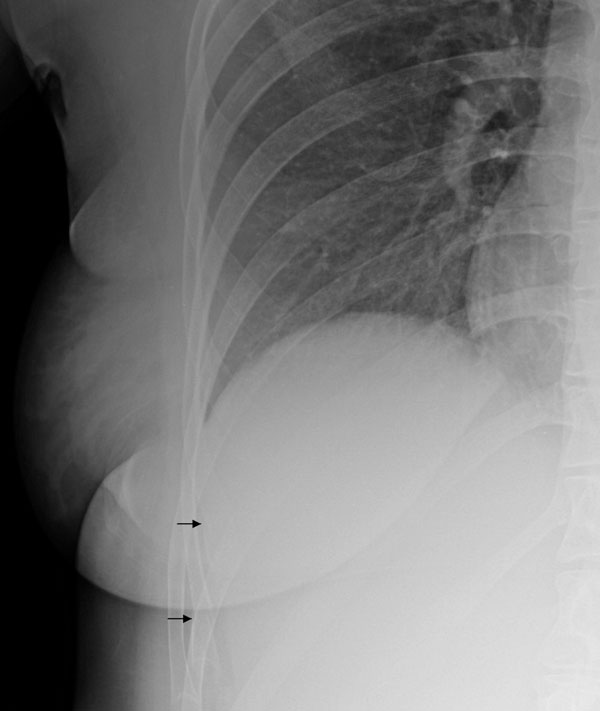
Right rib cage X-ray of the patient showing fractures of the 8^th^ and 9^th^ rib.
